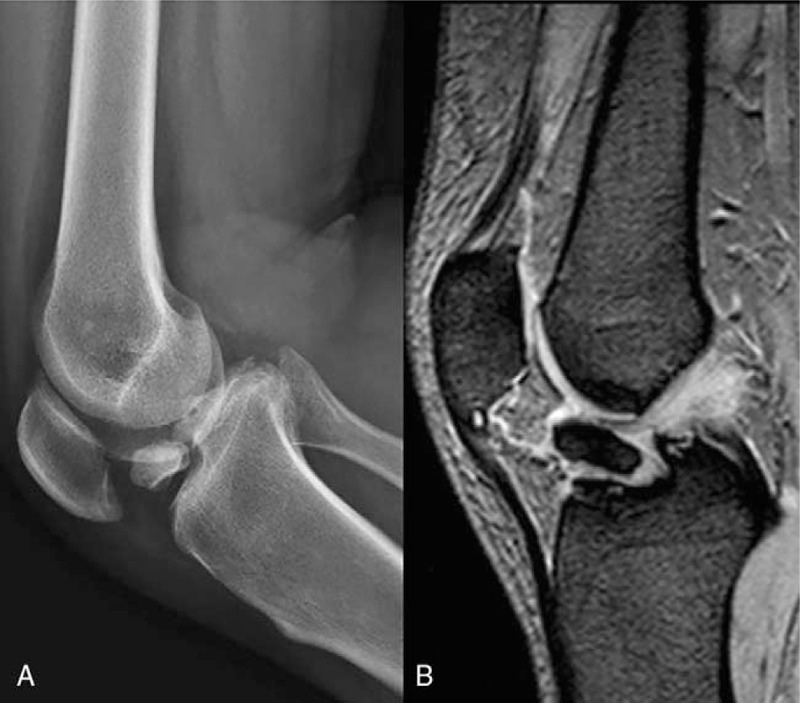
Preoperative radiograph and MRI findings in a case of ununited tibial eminence fracture. MRI = magnetic resonance imaging.
